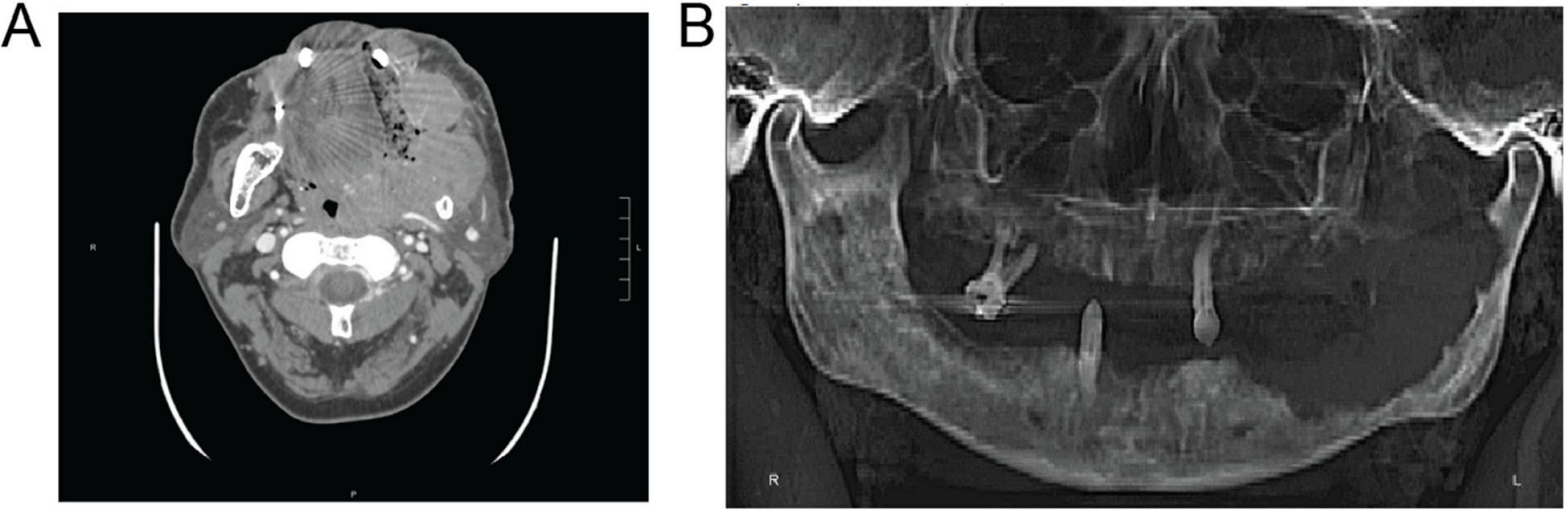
Imaging of a large squamous cell carcinoma of the oral cavity. A Cross sectional CT image demonstrating a 6.8 × 8.0 × 7.5 cm vascular soft tissue mass originating in the alveolar ridge of the left mandibular ramus with significant bony destruction. B X-ray prior to full mouth dental extraction showing 3 remaining teeth with gross caries and bony destruction of the left mandible
